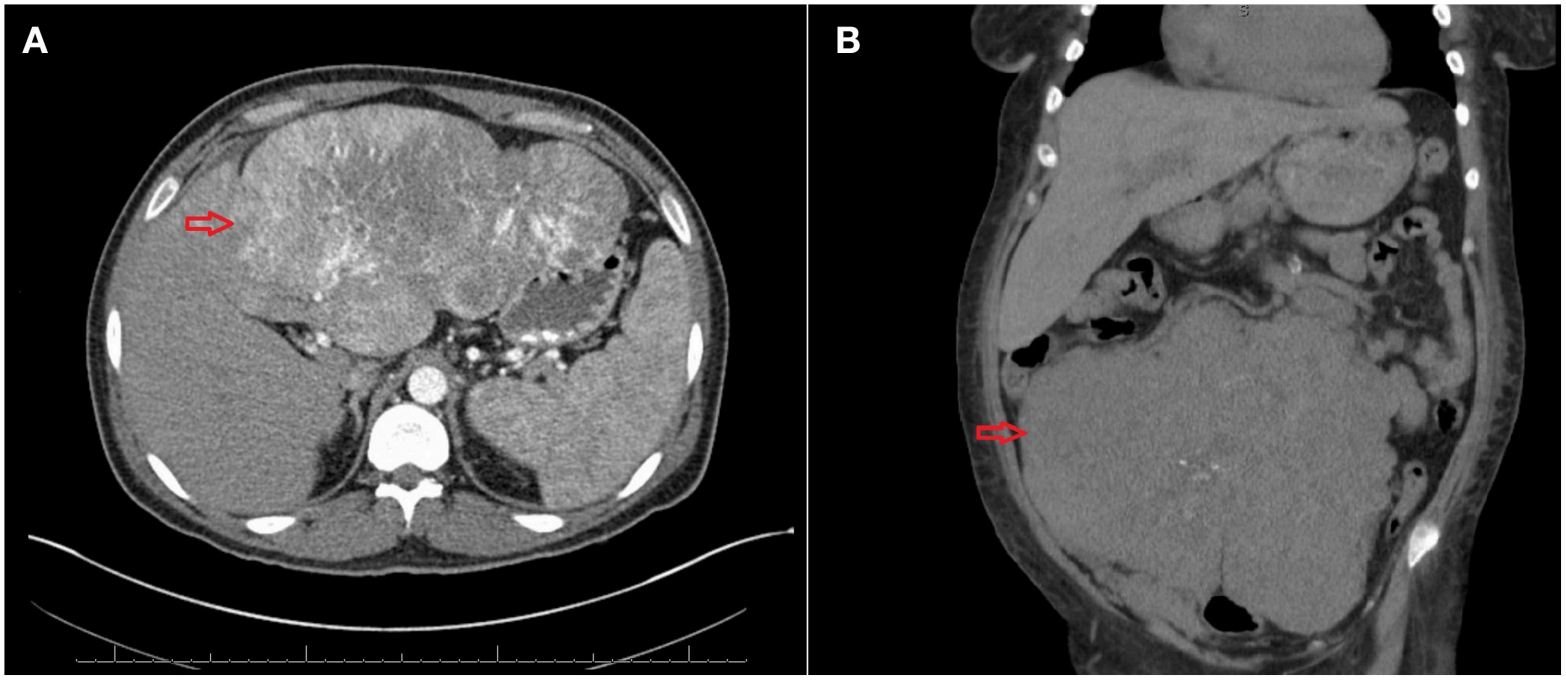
(A) Computed tomography (CT) of the abdomen showing a large mass of 20 cm size (red arrow). (B) CT of the abdomen showing a large tumor of 24 cm^*^ 18 cm^*^12 cm (red arrow).
